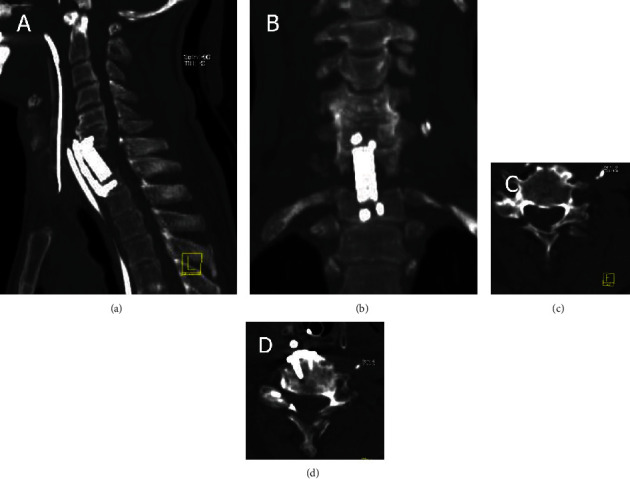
2D multiplanar CT reformation of the cervical spine in the bone window algorithm. (a, b) Sagittal and coronal views depict the location of plates and screws at the C6-T1 level. (c) Axial view above the fixation level. (d). Axial view at the T1 level.
